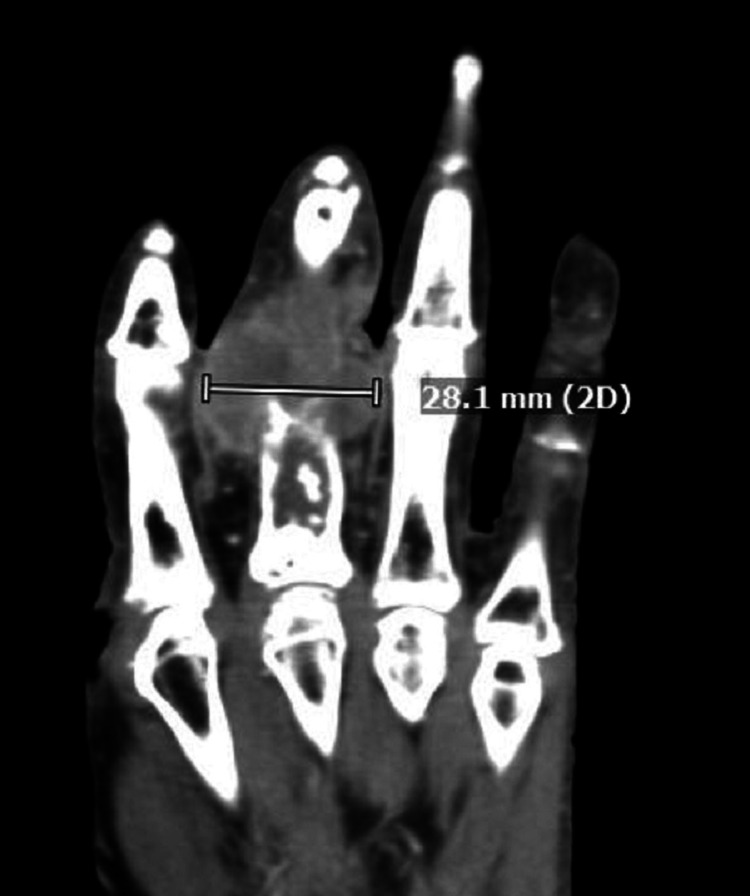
CT scan showing pathologic fracture in the distal aspect of the third proximal phalanx by tumor with chondroid features and extra-osseous soft tissue extension CT: computed tomography
